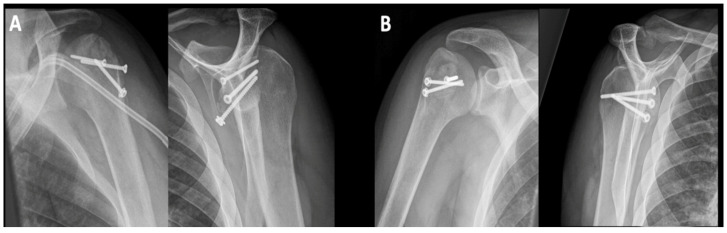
Postoperative imaging of the patient from Figure 1 and Figure 2; (A) left shoulder: 3-month postoperative X-rays of the left proximal humerus: no pain, no displacement of the screws (the opacification on the a.p. X-ray is a dialysis catheter); (B) right shoulder: 3-month postoperative X-rays of the left proximal humerus: no pain, no displacement of the screws.
